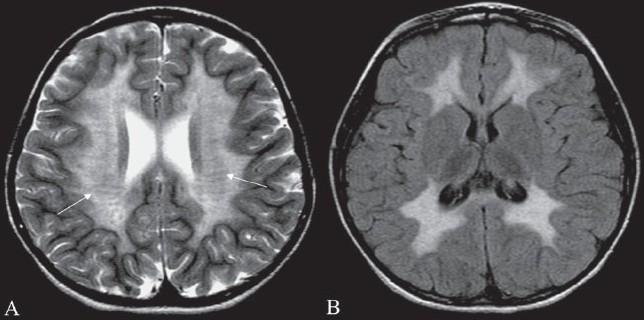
Tigroid pattern. Axial T2W image of the brain in a child with metachromatic leukodystrophy shows symmetric, increased signal intensity of the white matter, with sparing of the subcortical U fibers. Linear low signal intensity areas radiating away from the ventricular margin (arrows) represent areas of white matter around the vessels that have been spared from the process of demyelination. These low signal linear areas within the hyperintense white matter resemble the skin of a leopard and hence the term ‘tigroid’ pattern. FLAIR axial MRI image (B) shows symmetric hyperintensity of the white matter sparing the subcortical U fibers
